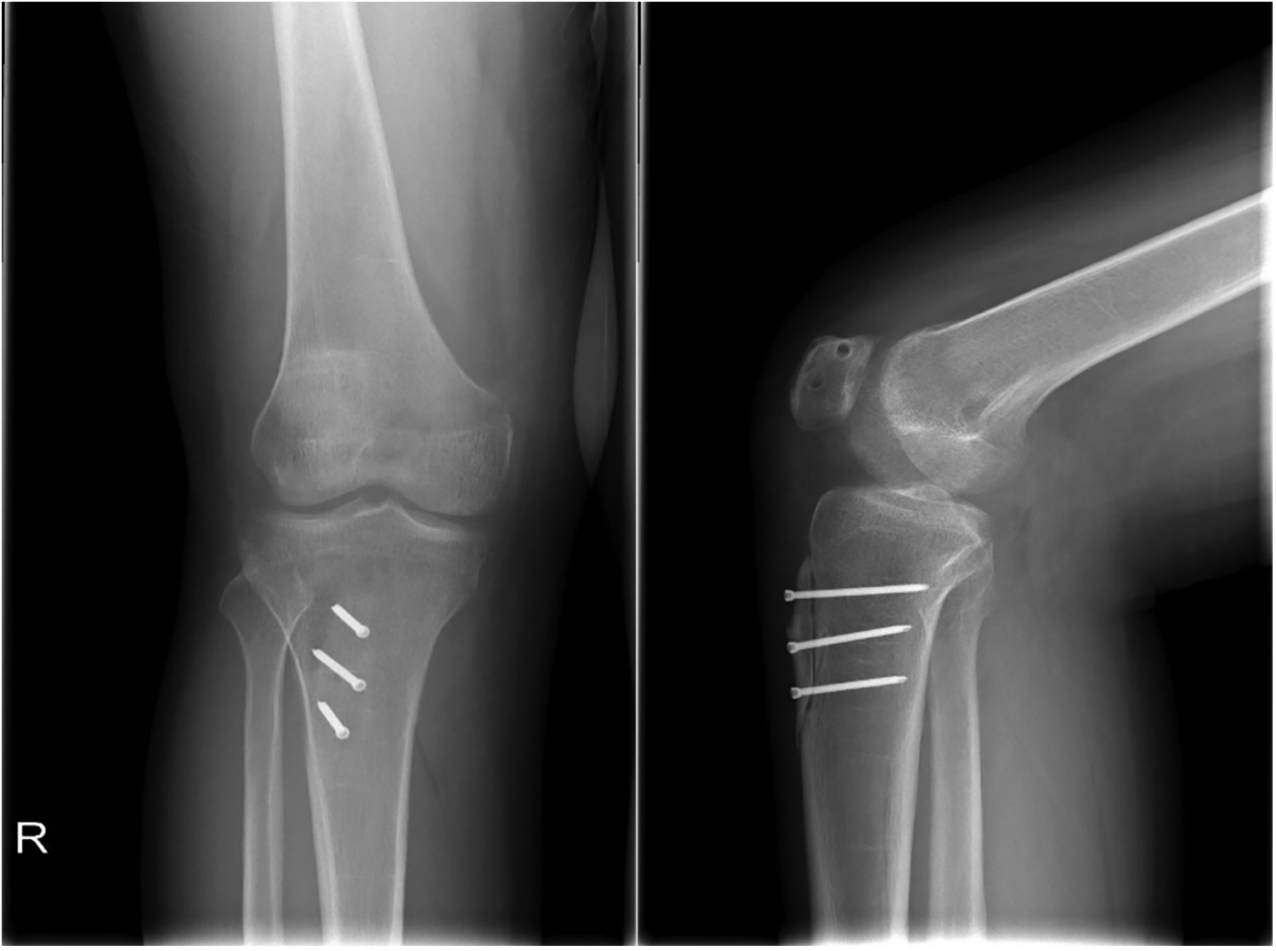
Radiograph after MPFLR combined with TTO. MPFLR, medial patellofemoral ligament reconstruction; TTO, tibial tubercle osteotomy.
